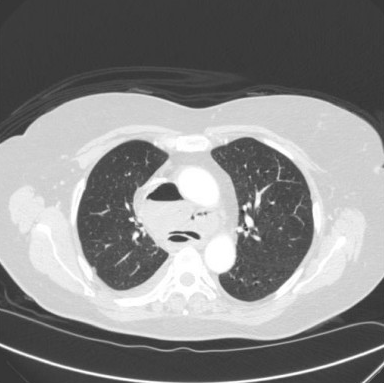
Contrast enhanced axial CT-scan of the thorax showing the cystic mass at the level of the carina, now with air-fluid levels, compare fig 2. Also shows bilateral pleural effusions worse on right side (lung window setting).
